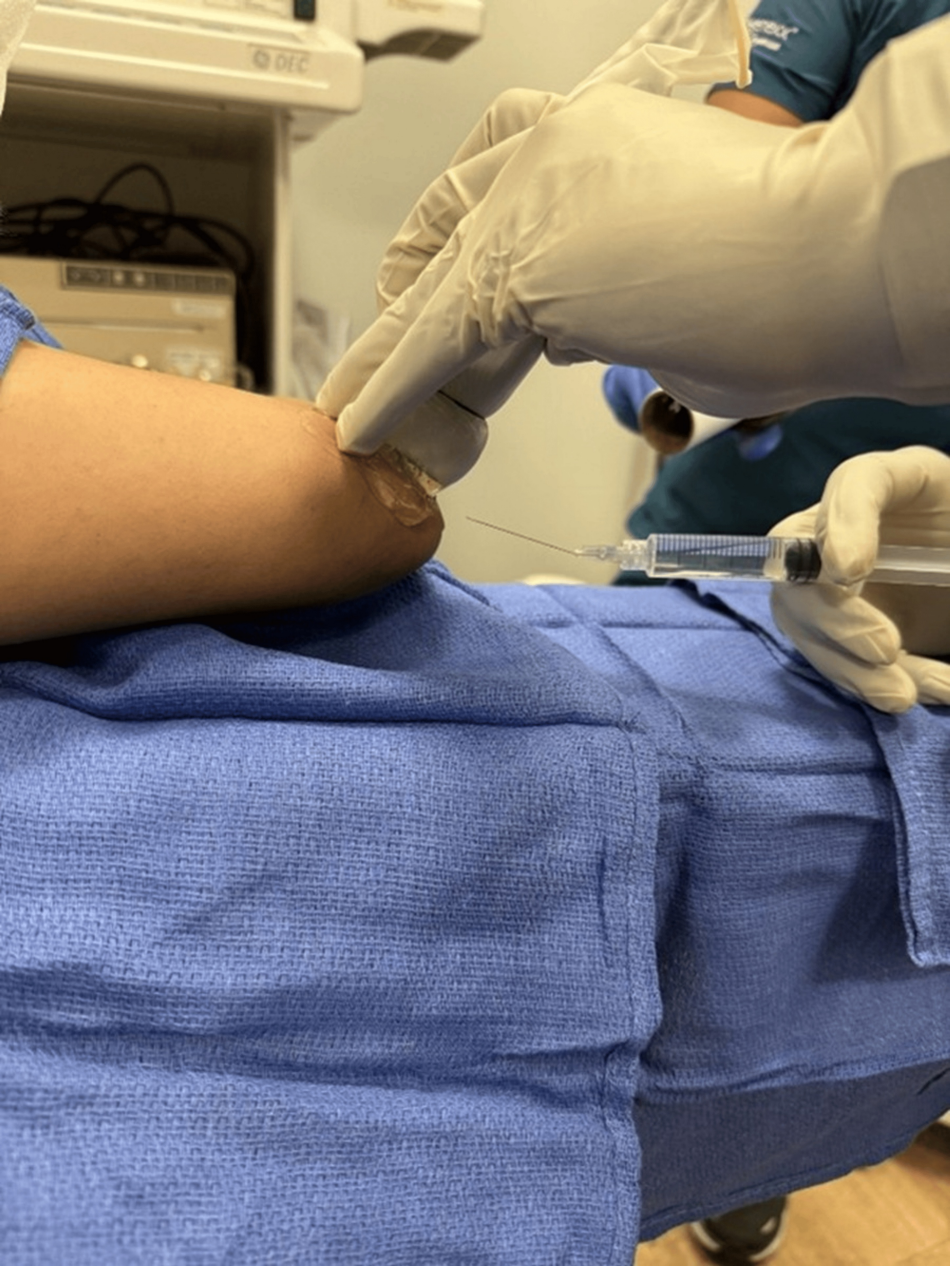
Ultrasound position and needle entry
